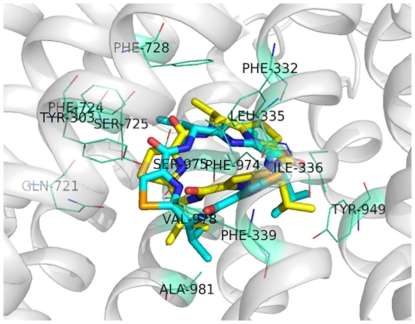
The Docking of QZ59-RRR to P-gp. Docking of QZ59-RRR to the X-ray structure of P-gp-QZ59-RRR complex (PDB code: 3G60) was performed using the Autodock Vina algorithm to validate the accuracy of docking calculations. The geometry of P-gp-QZ59-RRR complex obtained by docking calculations (yellow carbons) was compared with that of the P-gp-QZ59-RRR complex in the X-ray structure (blue carbons). Potential binding residues of P-gp for QZ59-RRR in the internal cavity are indicated.
